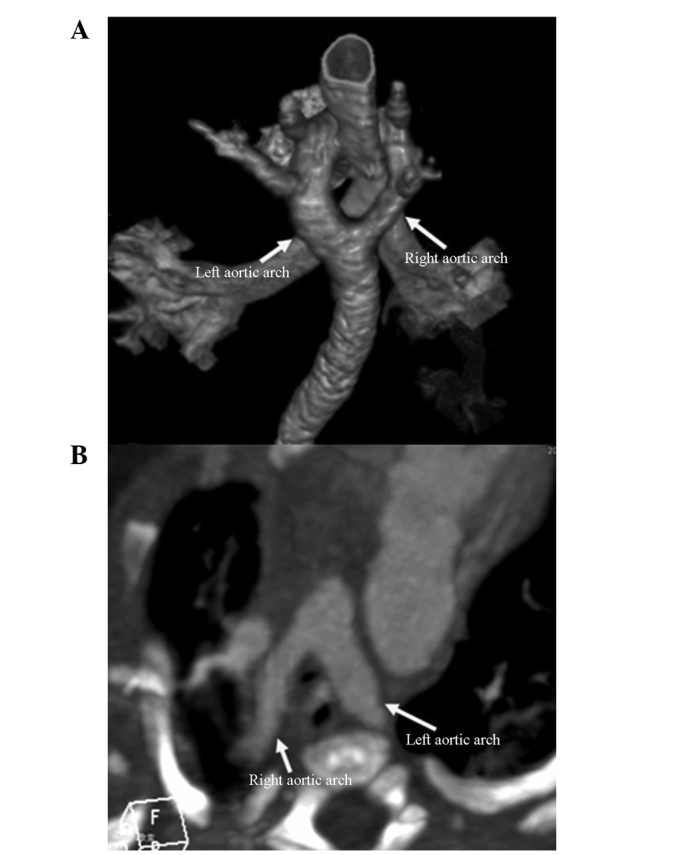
A four-month-old male infant with DAA. (A) The CT volume-rendered image shows that the aortic arch split into two arches surrounding the trachea (thick left arch and small right arch). (B) An axial CT section image. The aortic arch split into two arches surrounding the trachea and esophagus. DAA, double aortic arch; CT, computed tomography.
